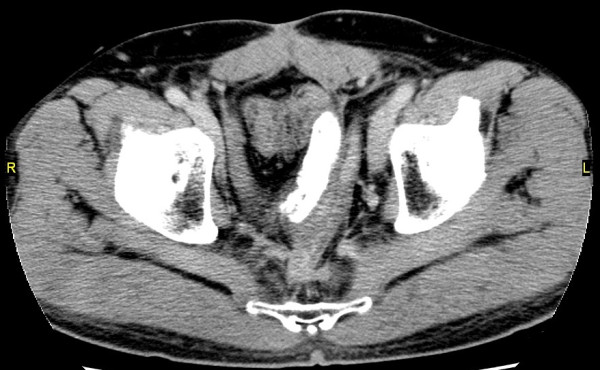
CT scan showed spacer placed between the tumor and small bowel.
